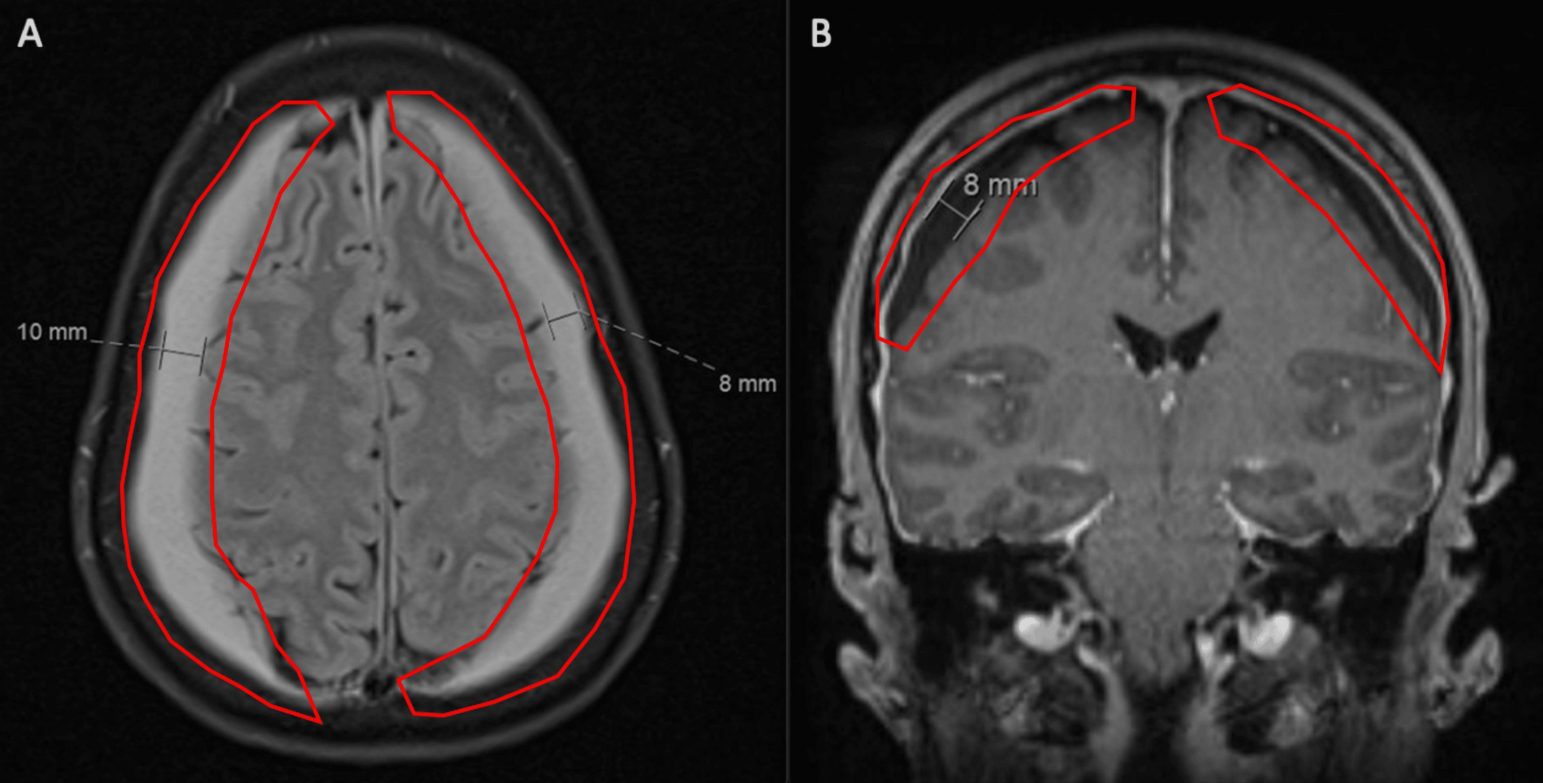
Axial and coronal follow-up MRI scans. A) Axial follow-up T1-weighted MRI demonstrating stigmata of intracranial hypotension, including minimal worsening of pachymeningeal enhancement and thickening (red). The sizes of the collections increased to 10 mm on the right and 8 mm on the left. B) Coronal follow-up T1-weighted MRI demonstrating stigmata of intracranial hypotension, including minimal worsening of pachymeningeal enhancement and thickening (red).
